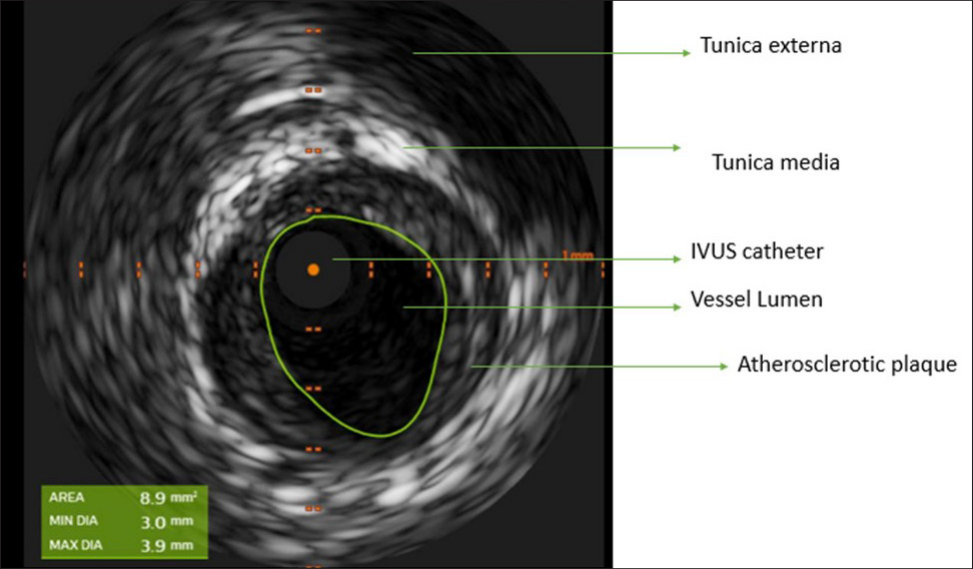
Intravascular ultrasound (IVUS) of the left main artery showing minimal luminal area of 8.9 mm^2^ and mild-moderate plaque burden.
